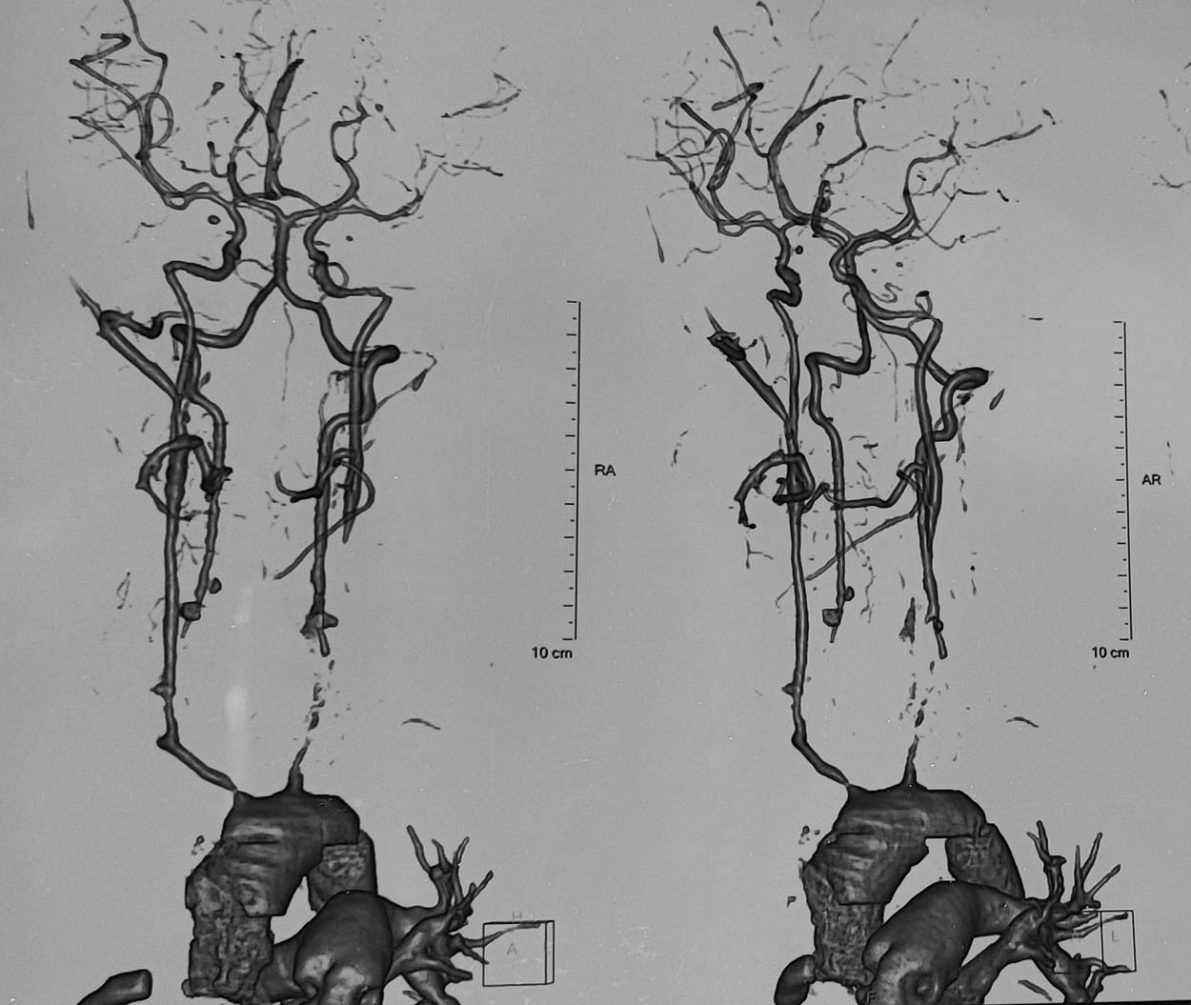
CTA showing severe focal narrowing of right ICA, marked wall thickening of the whole length of the left CCA with severe stenosis.
